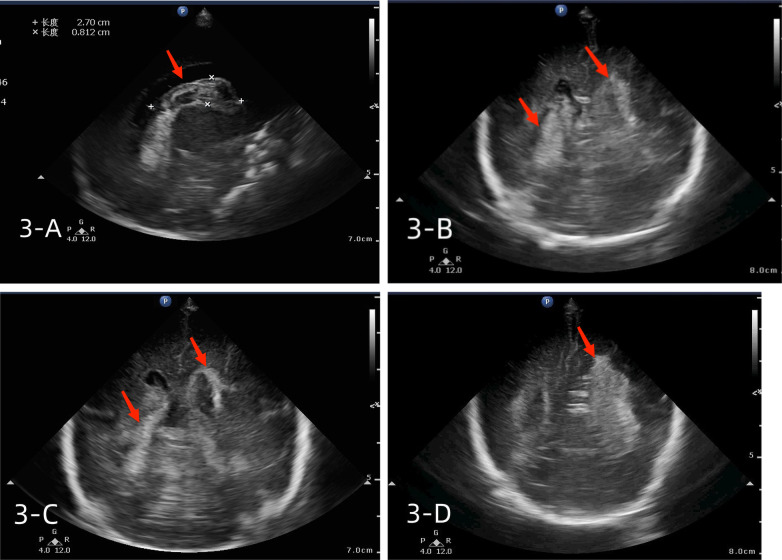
Cranial ultrasound in severe intraventricular hemorrhage. (A) Sagittal view showing dilated ventricles filled with echogenic blood (red arrows). (B–D) Coronal views demonstrating bilateral ventricular hemorrhage (red arrows) and periventricular echogenicity.
